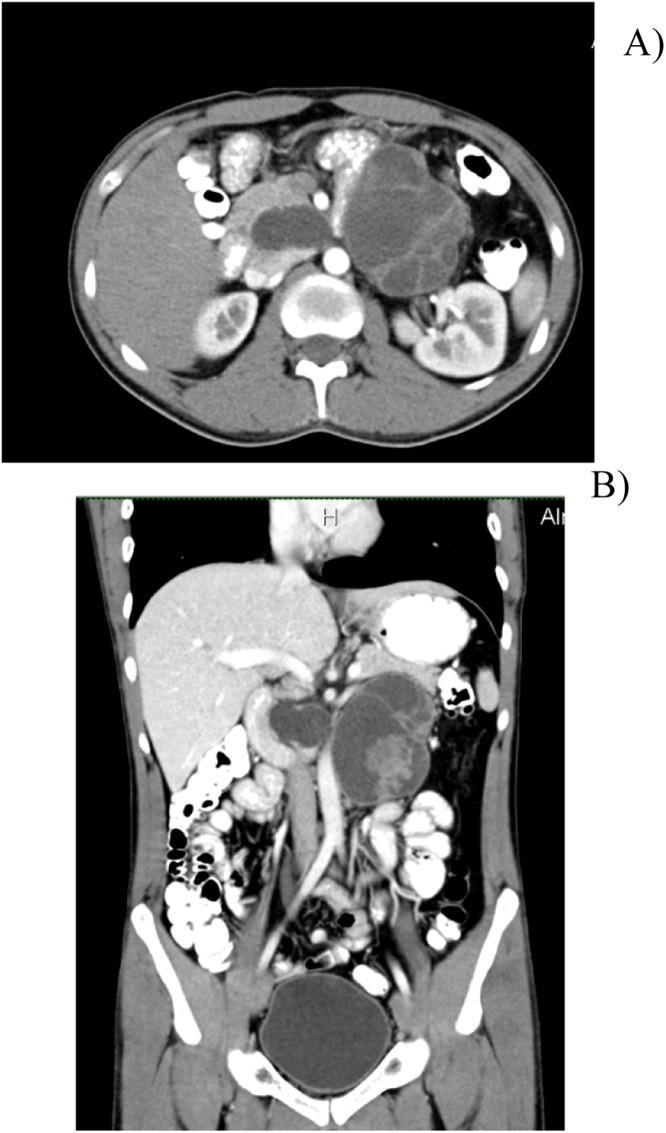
A) Abdominopelvic CT scan with I/V contrast report showing 2 lesions in the pancreas. B) Coronal section demonstrates the cystic lesion with IV contrast.
